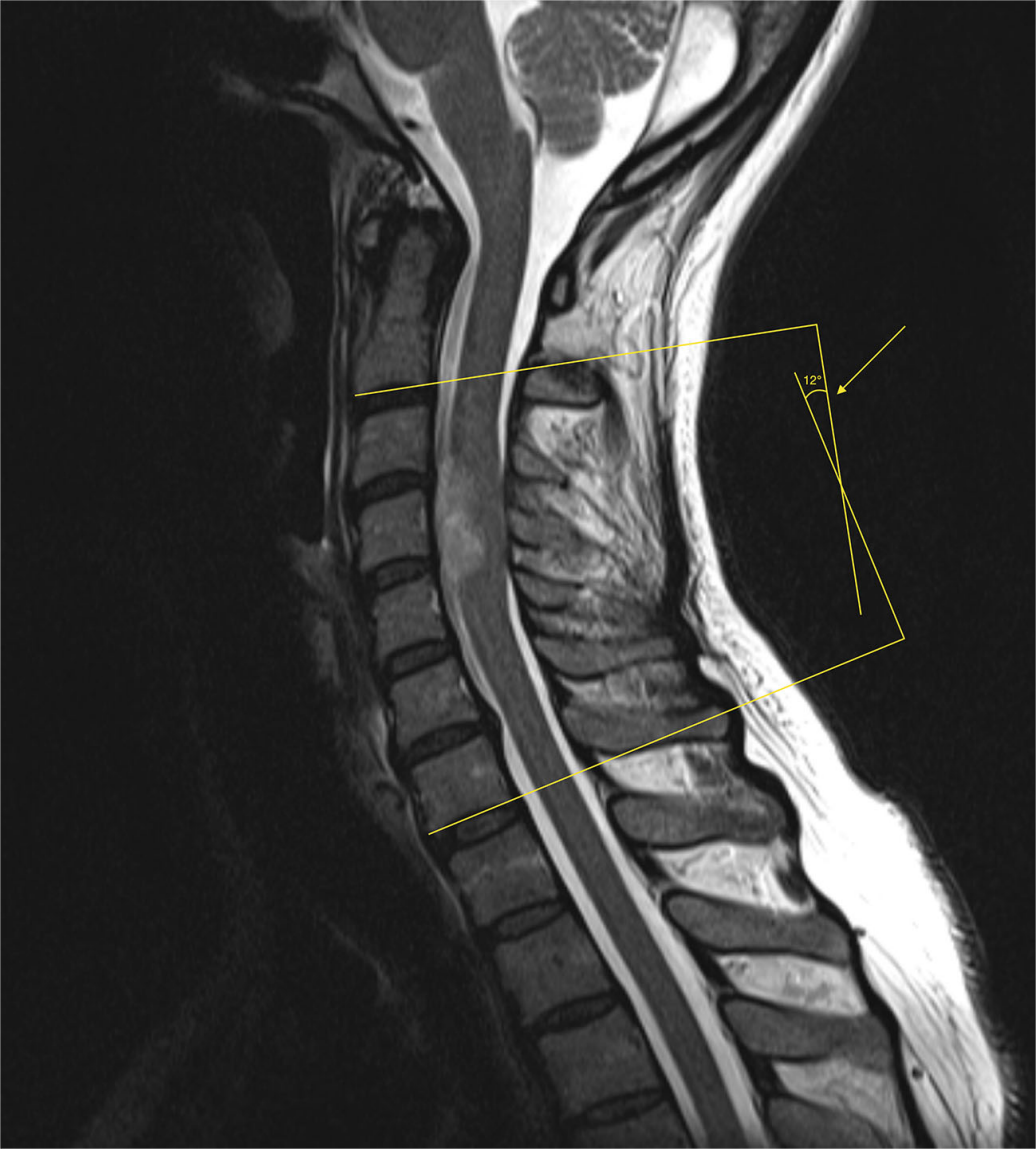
Measurement of a 12° C2–C7 Cobb angle from a sagittal T2-weighted magnetic resonance image
